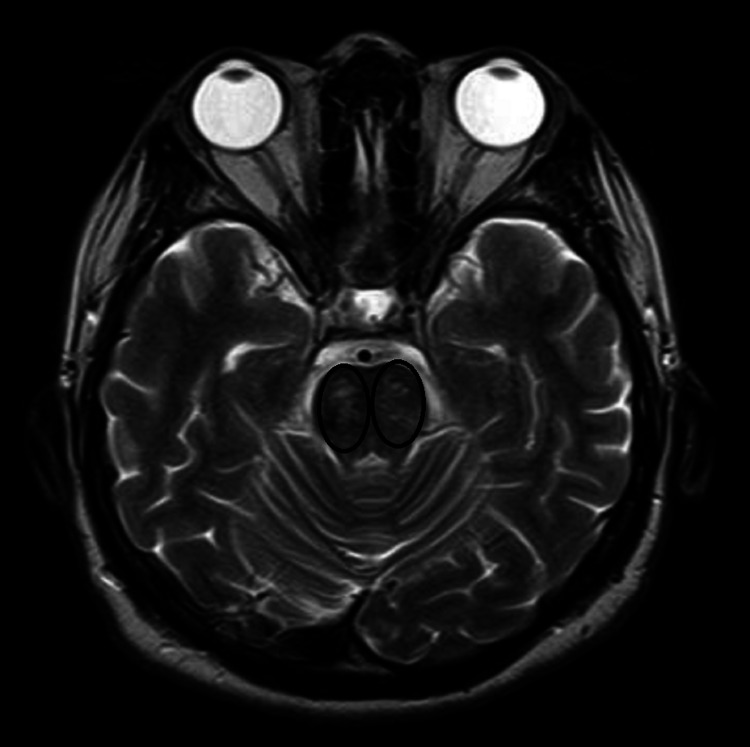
T2/FLAIR axial MR image showing hyperintense lesion over bilateral pons (marked by black circles) FLAIR: Fluid-attenuated inversion recovery
